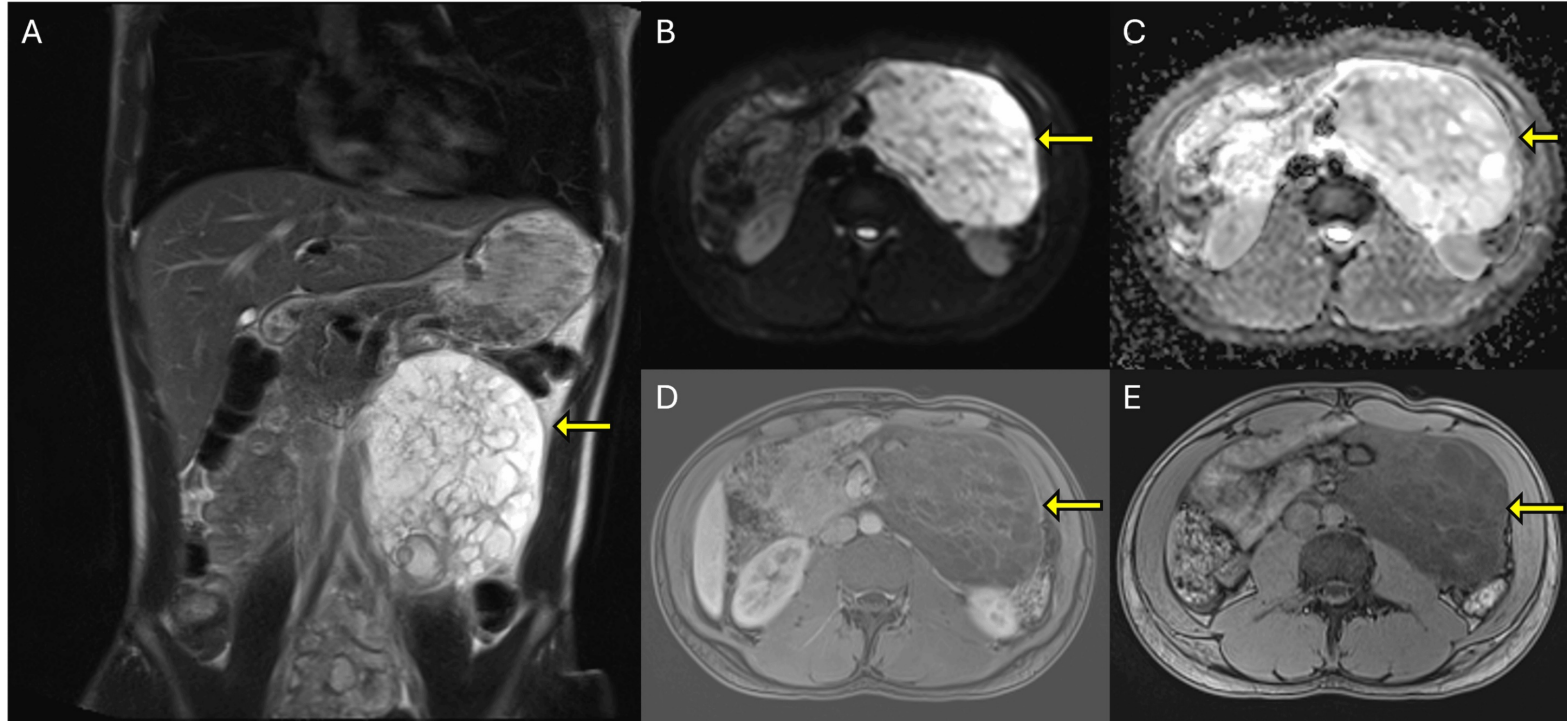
MRI characterization of the abdominal mass. (A) Coronal T2-weighted image demonstrates a large, multiloculated, hyperintense mass with internal septations in the left abdomen. (B) DWI shows high signal intensity within the lesion. (C) Corresponding ADC map demonstrates no true diffusion restriction, consistent with T2 shine-through. (D) Axial post-contrast fat-suppressed T1-weighted image shows mild enhancement of internal septations without solid enhancing components. (E) Axial T1-weighted image shows the lesion as hypointense relative to the surrounding soft tissues. MRI: magnetic resonance imaging, DWI: diffusion-weighted imaging, ADC: apparent diffusion coefficient
